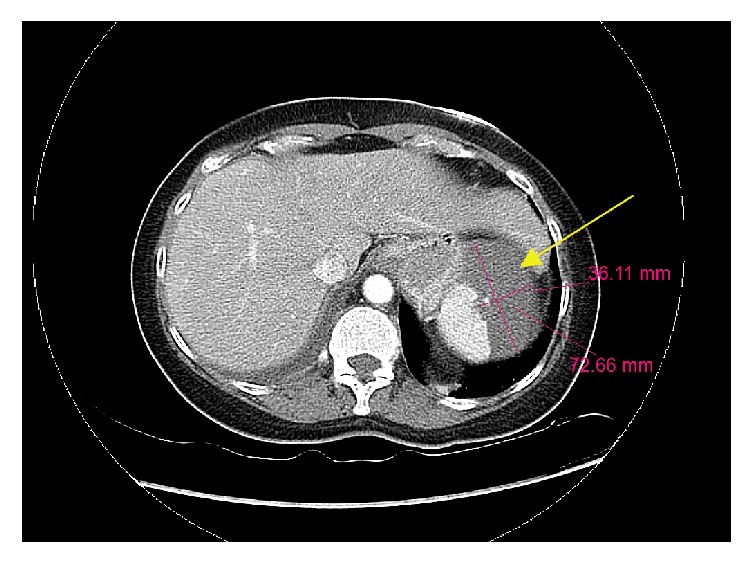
Computed Tomography (CT) of the abdomen done at admission with intravenous and oral contrast: the yellow arrow shows a high-density fluid filled large defect in the superior aspect of the spleen consistent with splenic infarct.
